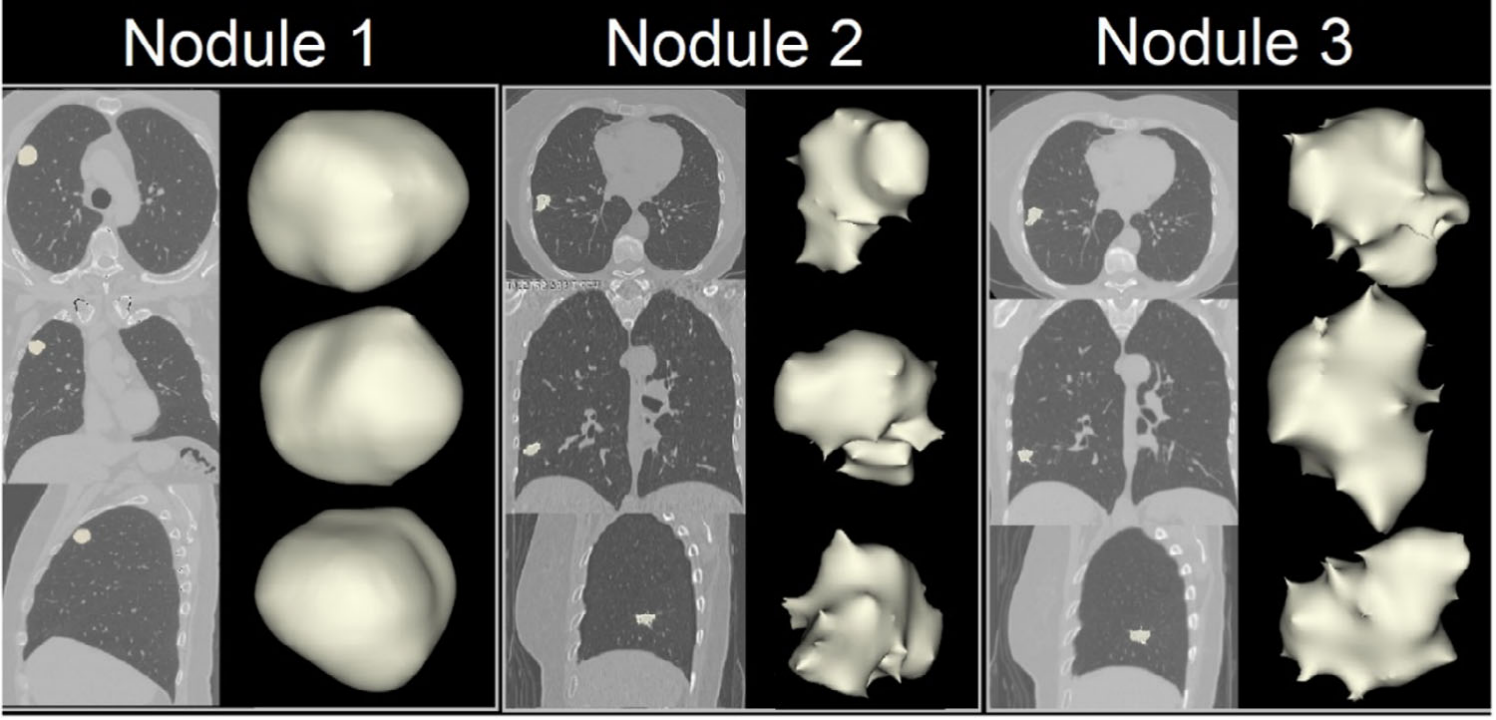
Lung nodules located in the patient's lung CT images and corresponding segmentations are to be printed. CT, computed tomography.
